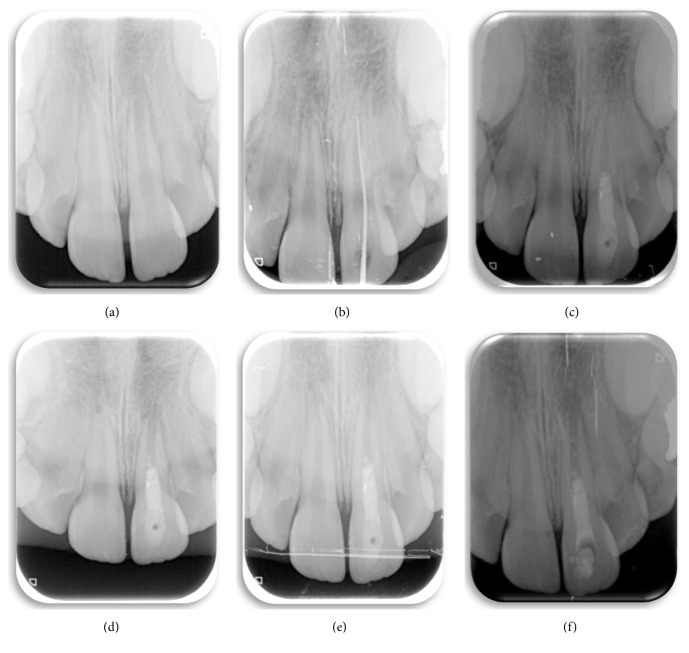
(a) Periapical radiograph image, 6 months after a trauma-induced subluxation of tooth 21, shows open apex of more than 2 mm, with periapical radiotranslucency. (b) Start of revascularization treatment of tooth 21, determination of working length. (c) Chamber sealed with MTA and wet cotton pellets, waiting for it to set hard. (d) Follow-up radiograph at 3 months, the periapical radiotranslucency at tooth 21 has disappeared. (e) Seven months after completion of revascularization therapy, dentinal wall thickening can be observed at 21, with the consequent narrowing of the apical foramen. (f) One year and 7 months after completion of revascularization treatment and compared with the periapical radiograph taken when revascularization treatment ended, there is a noticeable increase in root length, similar to the adjacent central incisor, thickening of the root walls, and a closed apex.
